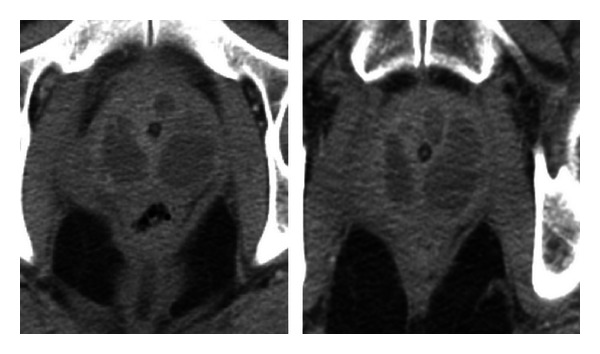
Showing the multiple prostatic abscesses on abdominopelvical CT.
